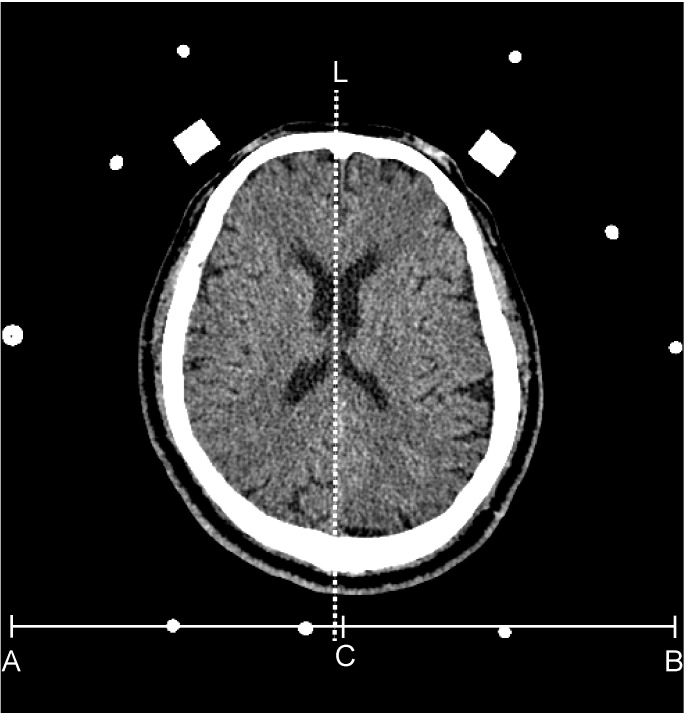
The dash line (L) indicates the position of the septum pellucidum in an axial view of a brain CT. The C point is the mid-point of the x-axis (from A to B) of the head frame. The distance between the dash line and point C is defined as the horizontal deviation.
